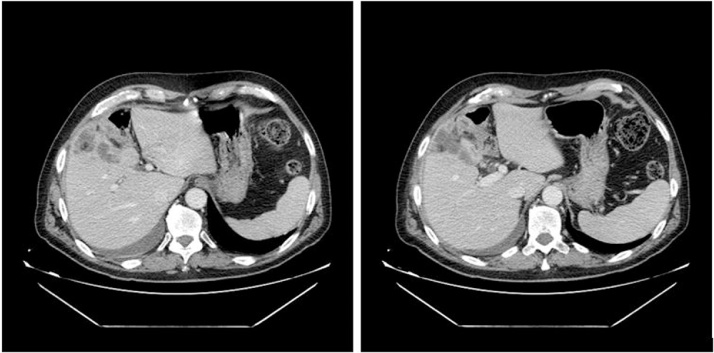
Abdominal CT scan shows a sclerotic gallbladder and right colonic flexure with walls harmonically thickened, with edema of the submucosa such as an inflammatory disease.
